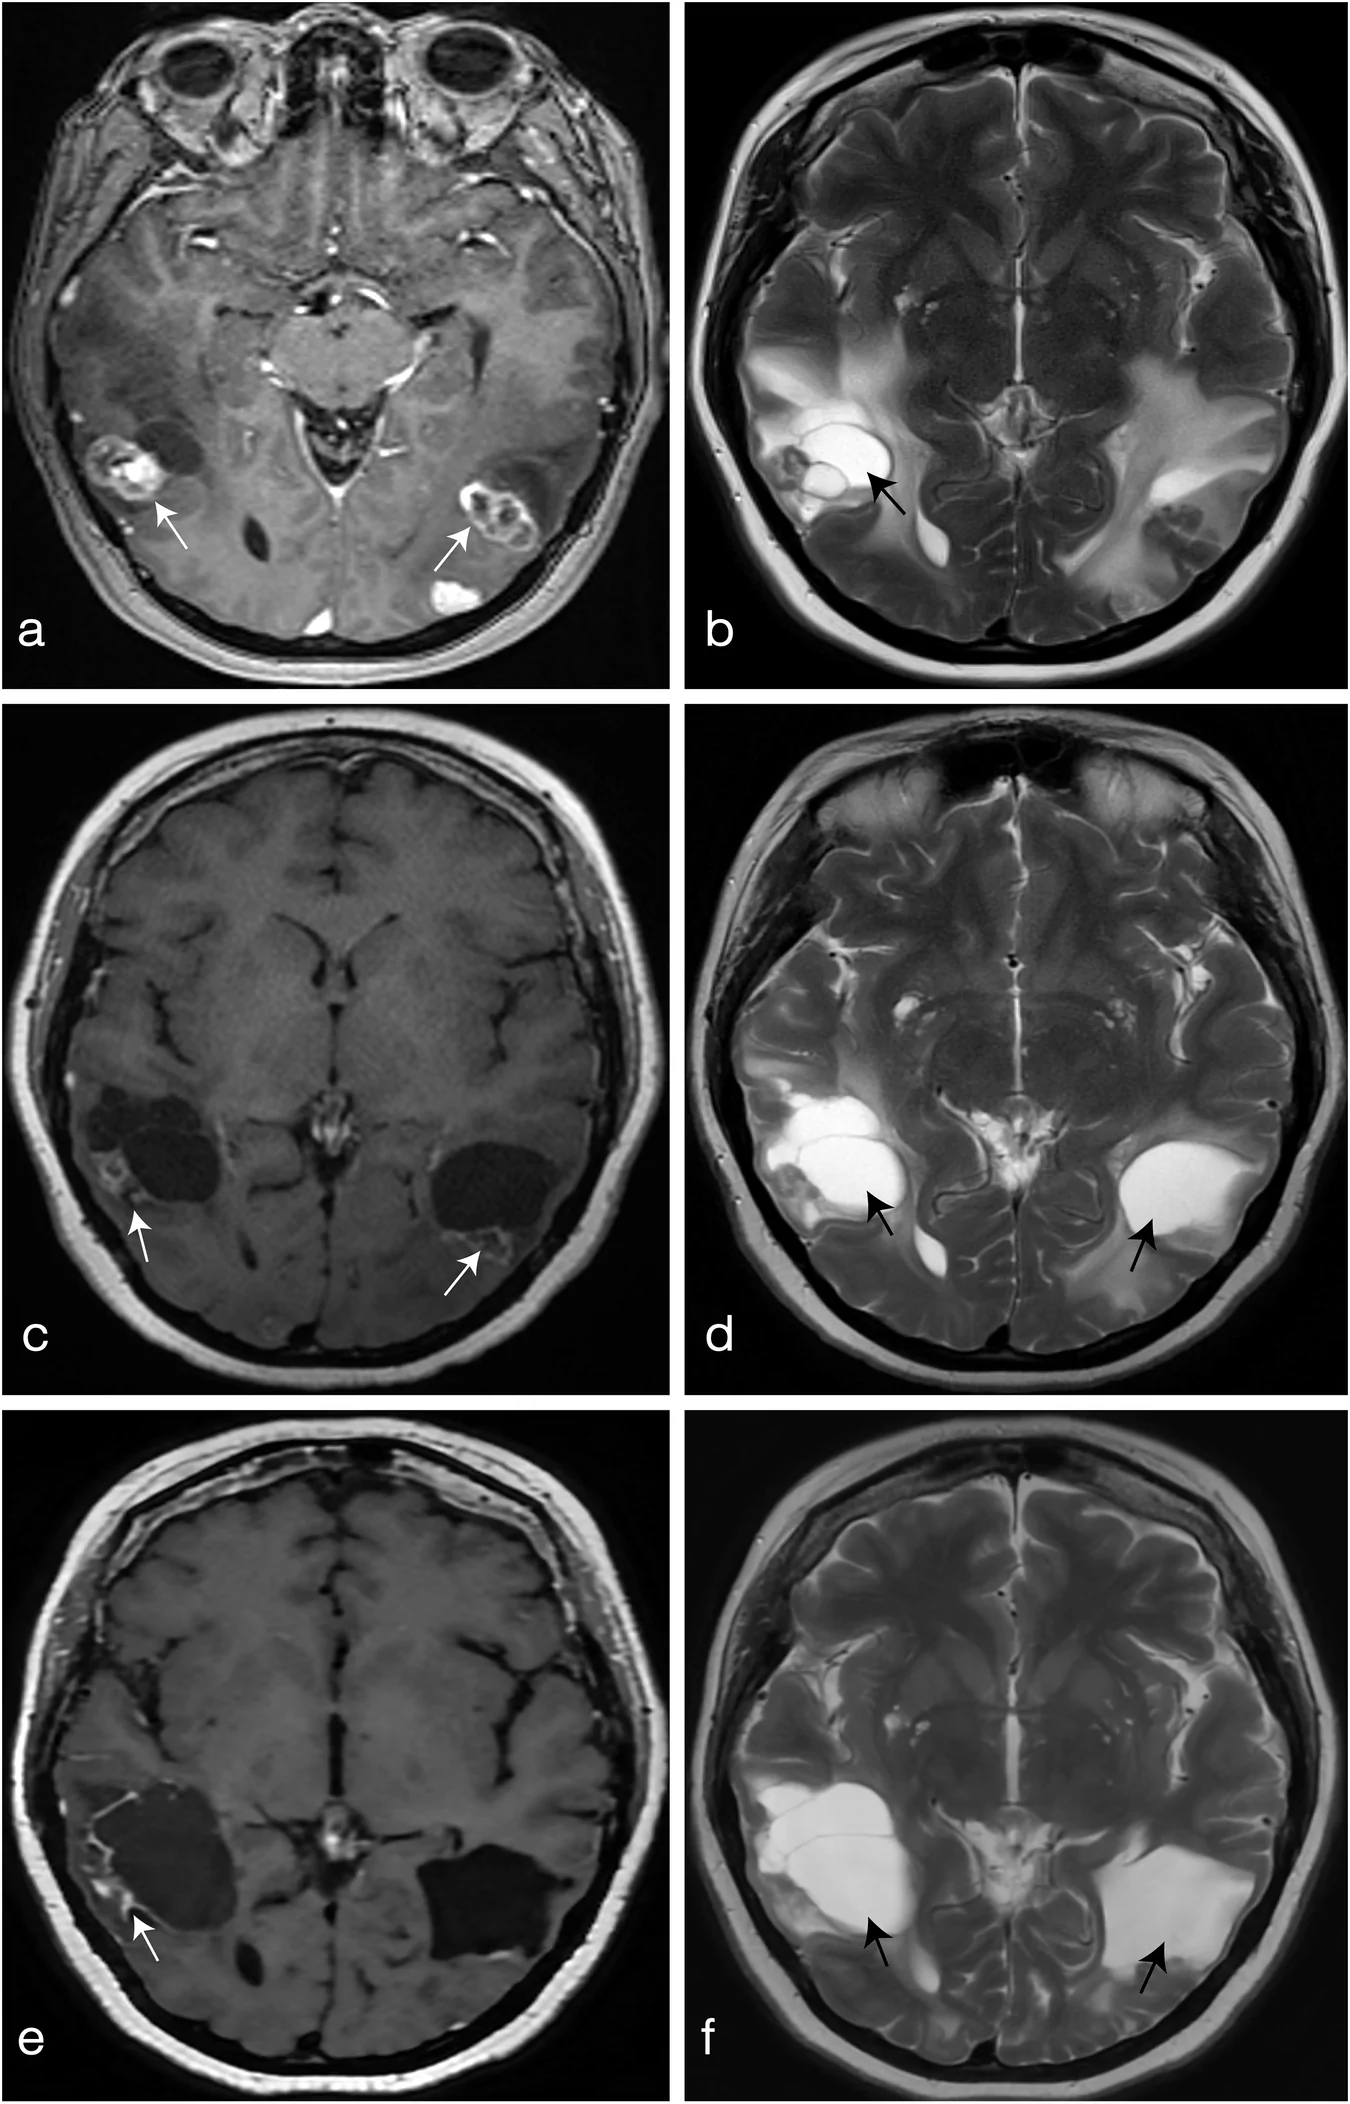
Progressive enlargement of the cystic component of brain metastases after stereotactic radiosurgery. A patient with metastatic breast cancer treated with stereotactic radiosurgery (SRS) for bilateral cerebral metastases. a, b Pre-SRS axial post-contrast T1-weighted (a) and T2-weighted (b) images showing bilateral enhancing solid-cystic cerebral metastases; white arrows in a mark the enhancing solid components, black arrow in b indicates the cystic component of the right cerebral lesion. c, d Three-month post-SRS images at the same level showing decreased size of the enhancing solid components (white arrows in c) and interval enlargement of the cystic components (black arrows in d). e, f Twelve-month post-SRS images showing further reduction/resolution of the enhancing solid components and progressive enlargement of the cystic components (white arrow in e; black arrows in f)
